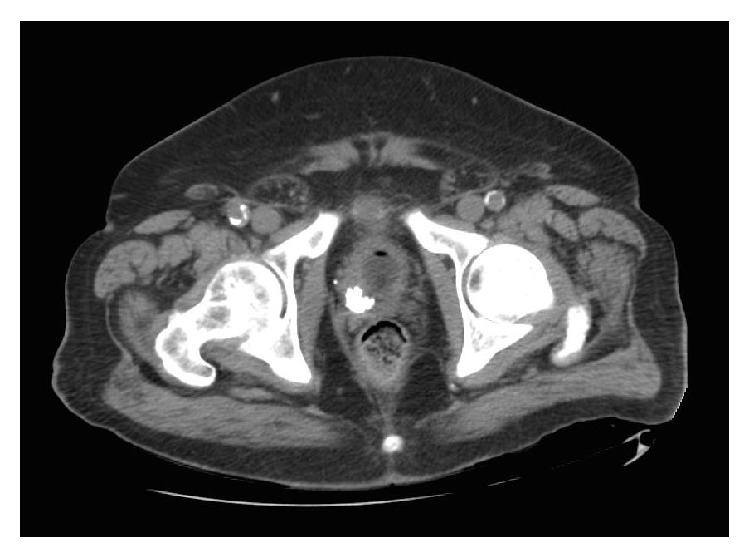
Axial image from the noncontrast pelvic CT scan demonstrating the right prostatic calcification measuring 17 × 12 mm in this section.
